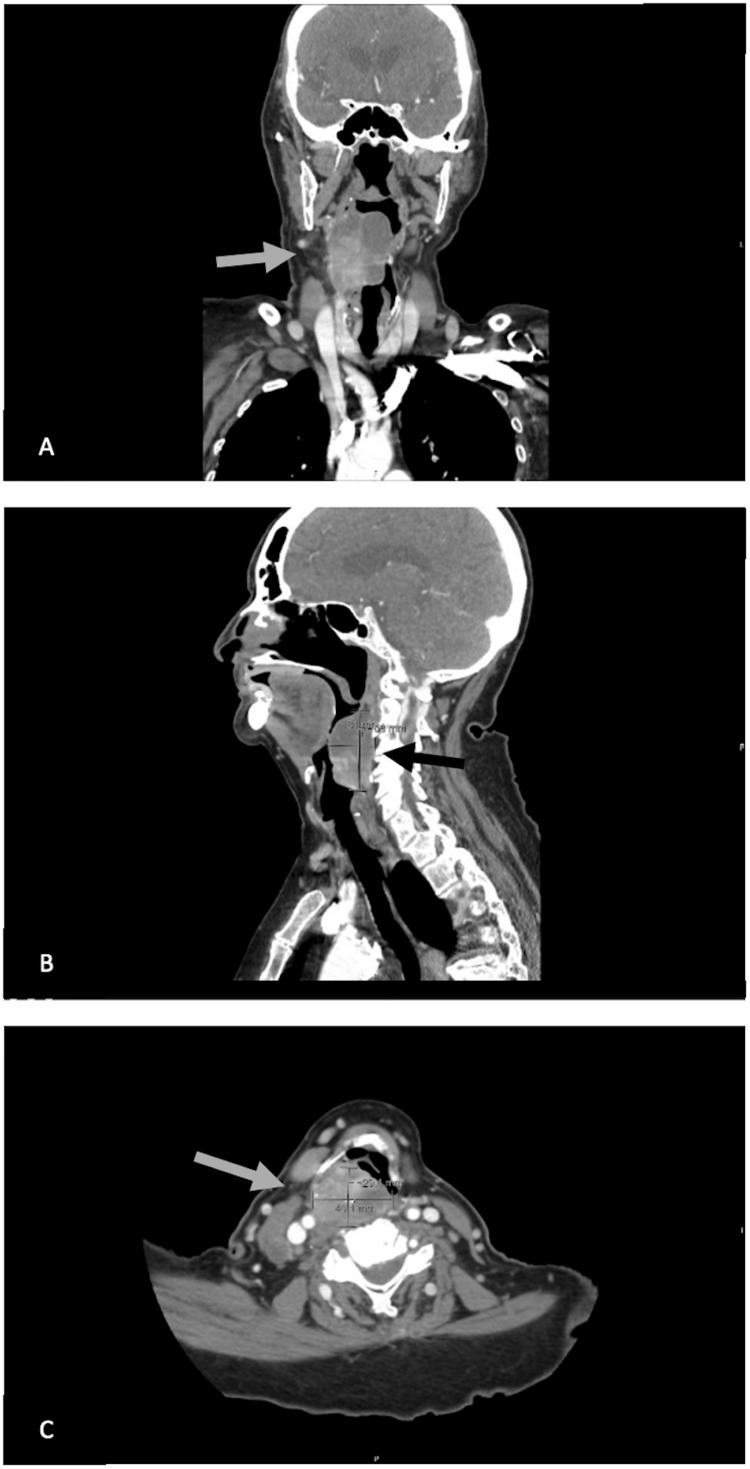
A CT scan demonstrating a 5x5x3 cm mass (indicated by arrows) superior to the larynx with lymphadenopathy in the (A) coronal, (B) sagittal, and (C) axial views. CT: Computed tomography
